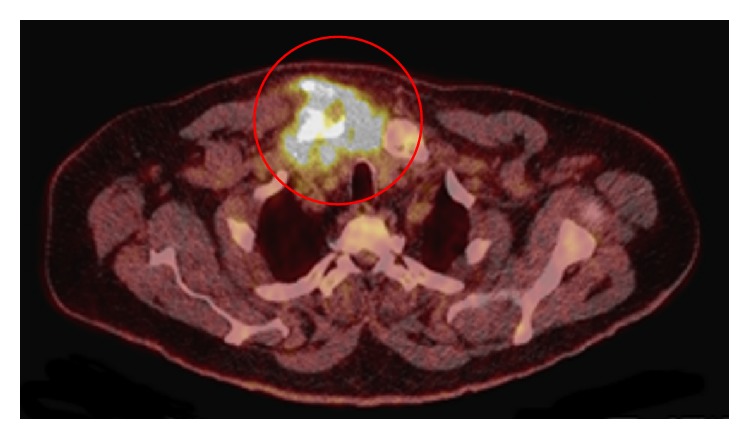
Patient 2. PET-CT cross-sectional view, with reaction in bone, joint, and nearby tissue.
